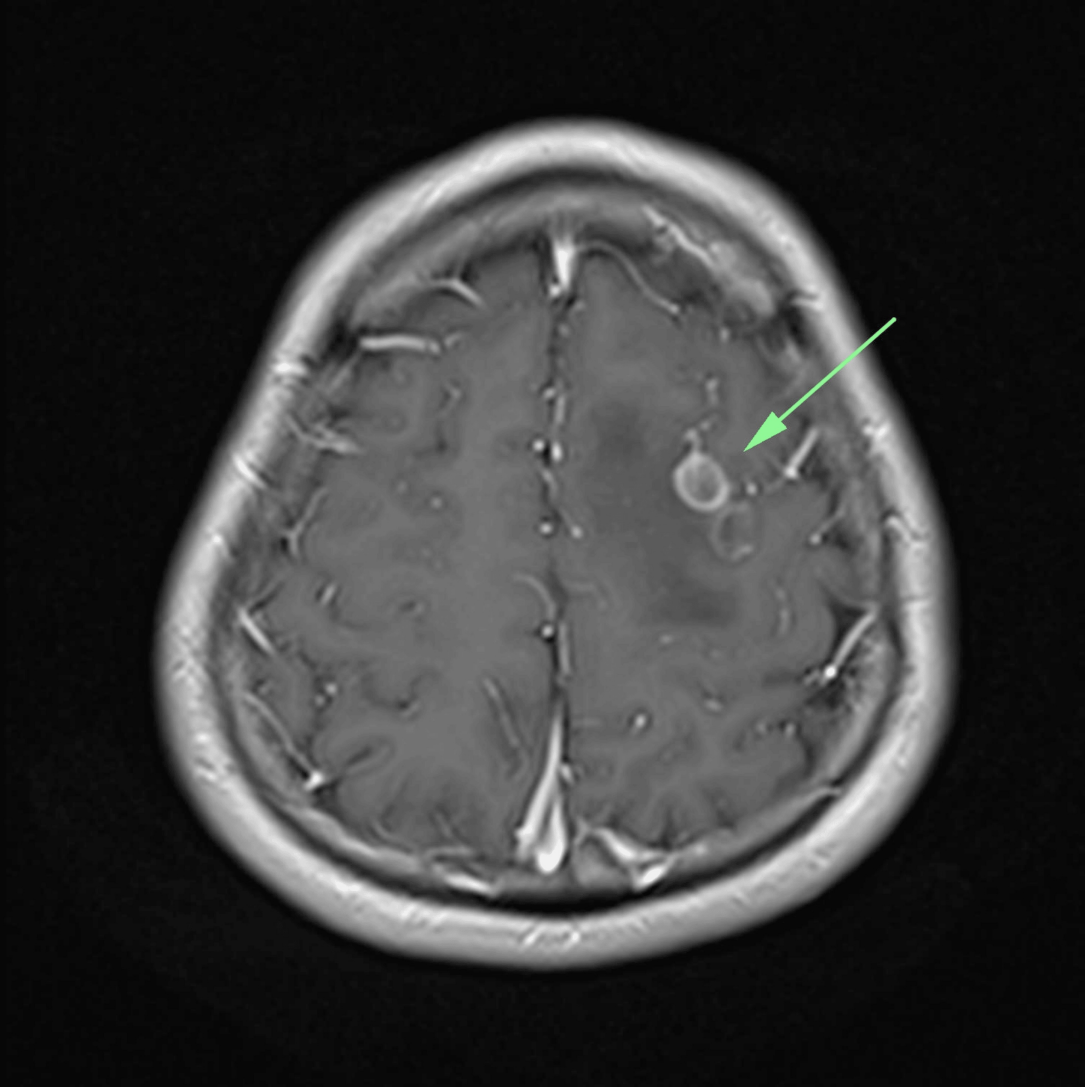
MRI of the brain, T1-weighted post-contrast axial section, showing left frontal cysts with varying degrees of peripheral enhancement and thickness of cystic wall (green arrow).
